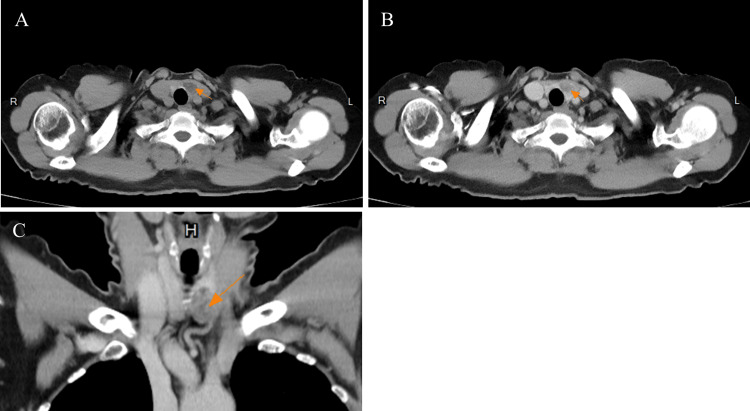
Chest CT with contrast of the left thyroid nodule A-C: The CT revealed a low-attenuation nodule measuring 2.5 x 1.7 x 1.2 cm in the left thyroid gland (orange arrow), with mild enhancement noted after contrast injection.
